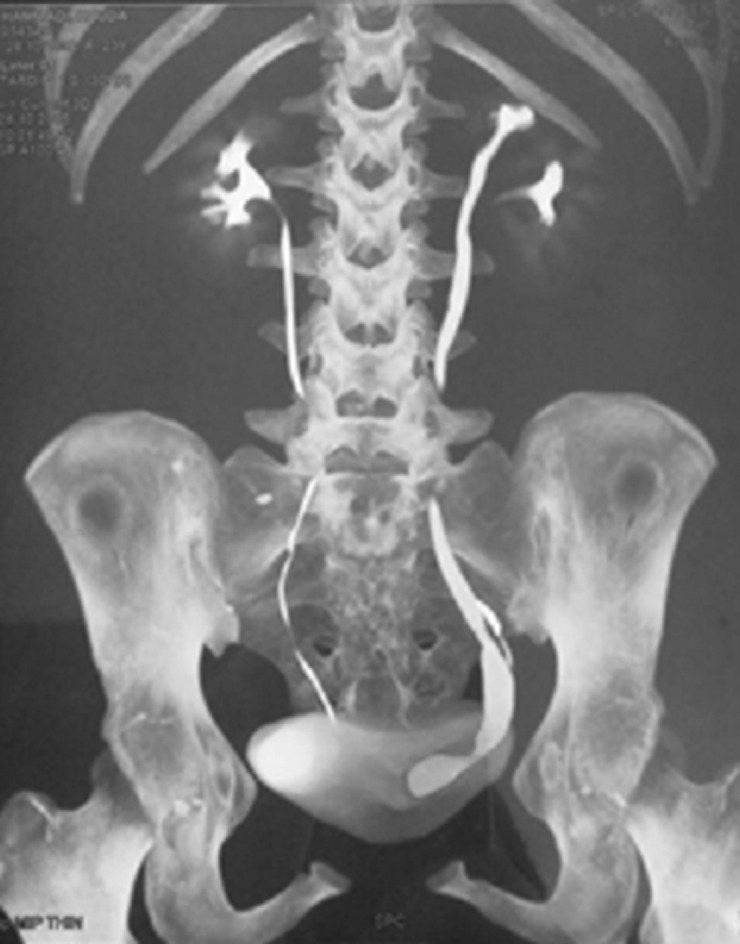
CT urography reconstruction layout of left ureterocele with left duplicated ureter at the expense of the upper renal lobule
